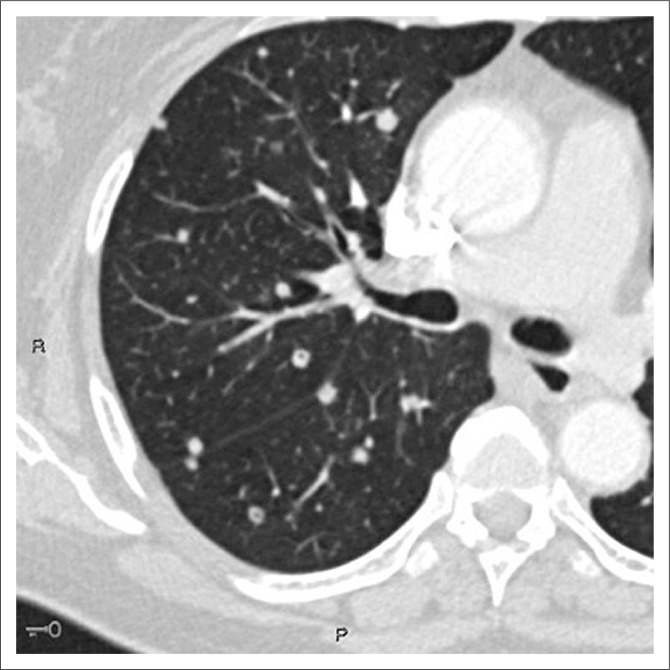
Computed tomography axial image through the right lung base demonstrates early central cavitation of the pulmonary nodules.
